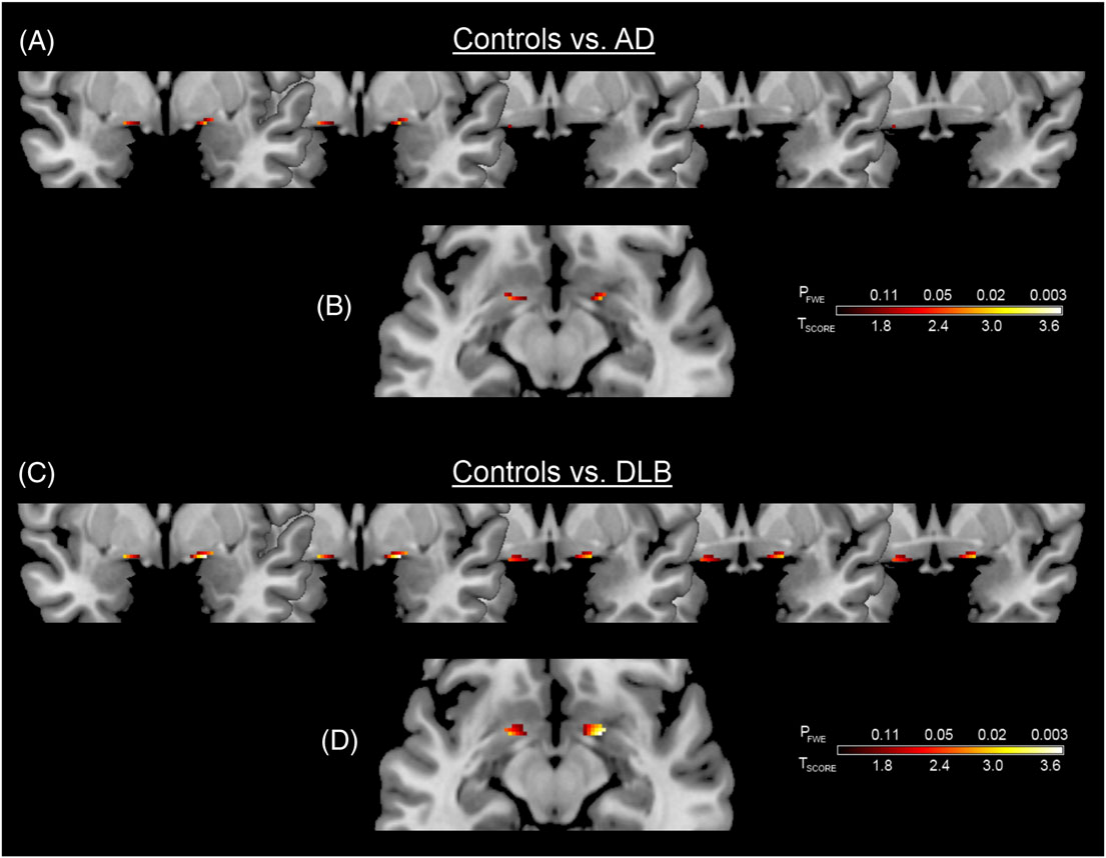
Significant grey matter loss of the substantia innominata in Alzheimer's disease (AD) and dementia with Lewy bodies (DLB) compared with controls. Results are superimposed on a magnetic resonance imaging T1 brain template image and displayed neurologically (left is left) in the coronal (A, C) and axial (B, D) views. [Colour figure can be viewed at wileyonlinelibrary.com]
